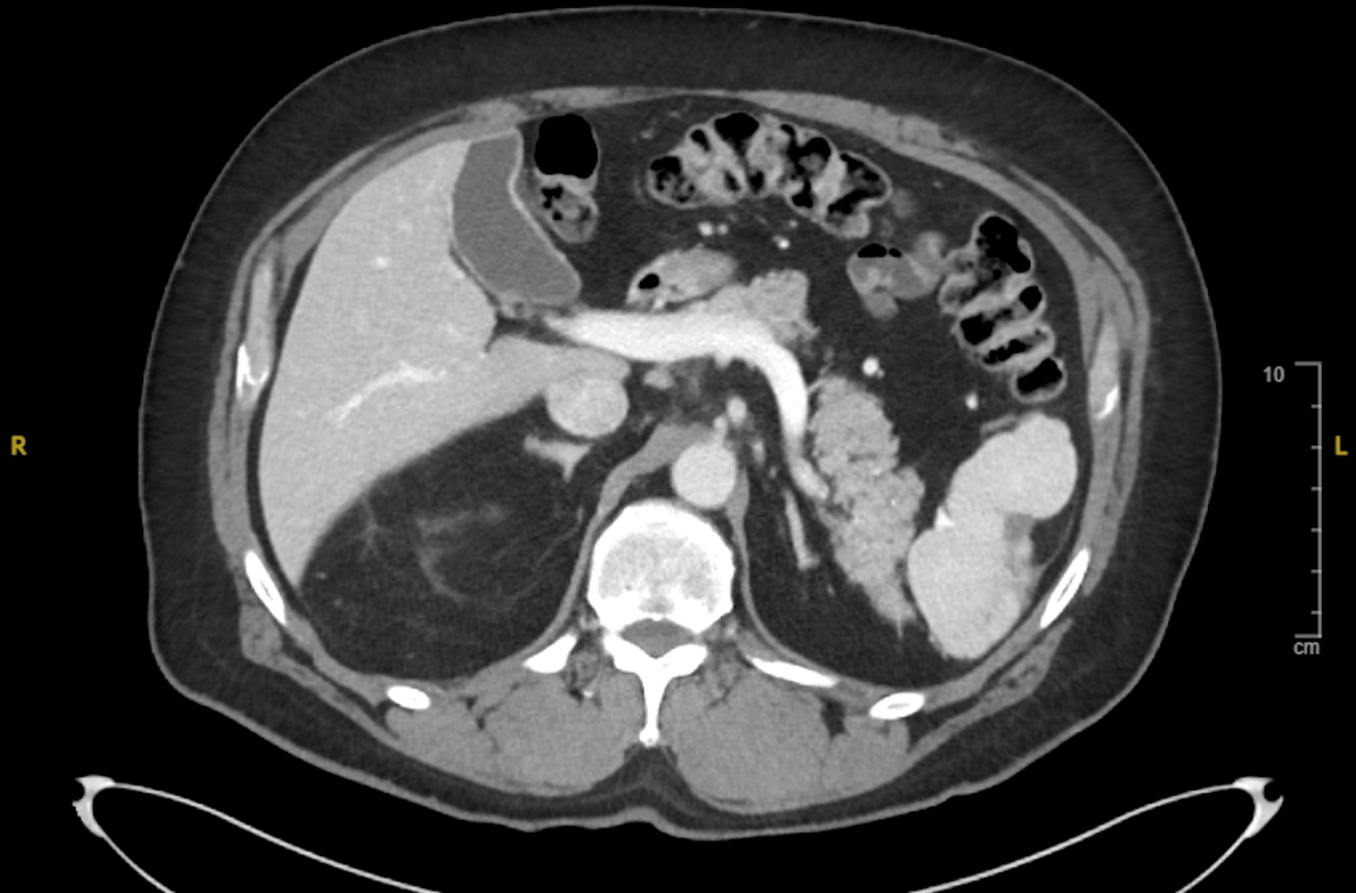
A CT scan of the abdomen with contrast demonstrated stable, evolving splenic infarcts without evidence of local tumor recurrence or new abdominal metastasis.
